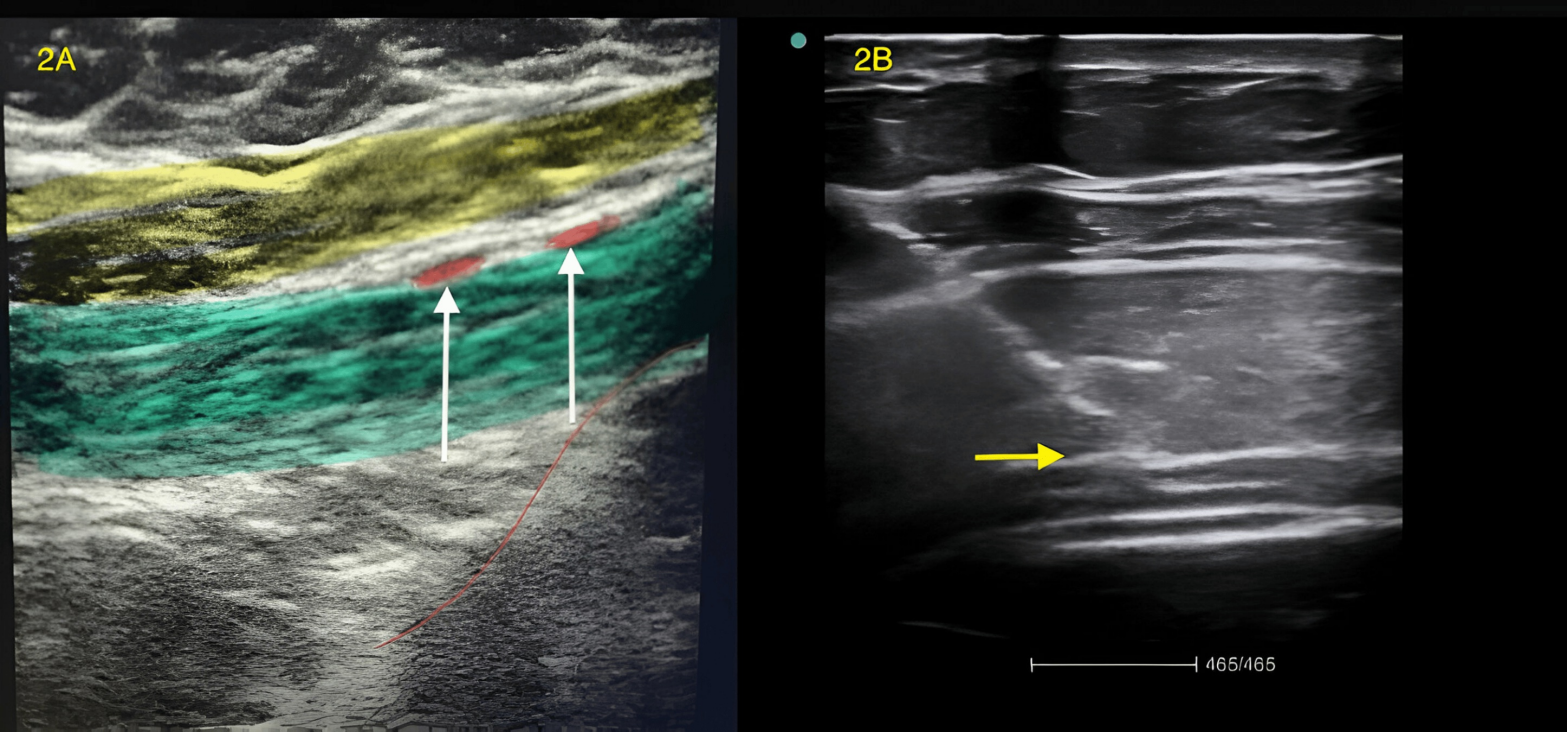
Ultrasound images showing Ilioinguinal, Iliohypogastric subcostal, and transversus abdominis plane block alone with intra-operative images. A: USG image of the ilioinguinal and iliohypogastric nerves (white arrows). B: Transversus abdominis plane block and the subcostal nerve (yellow arrow).
